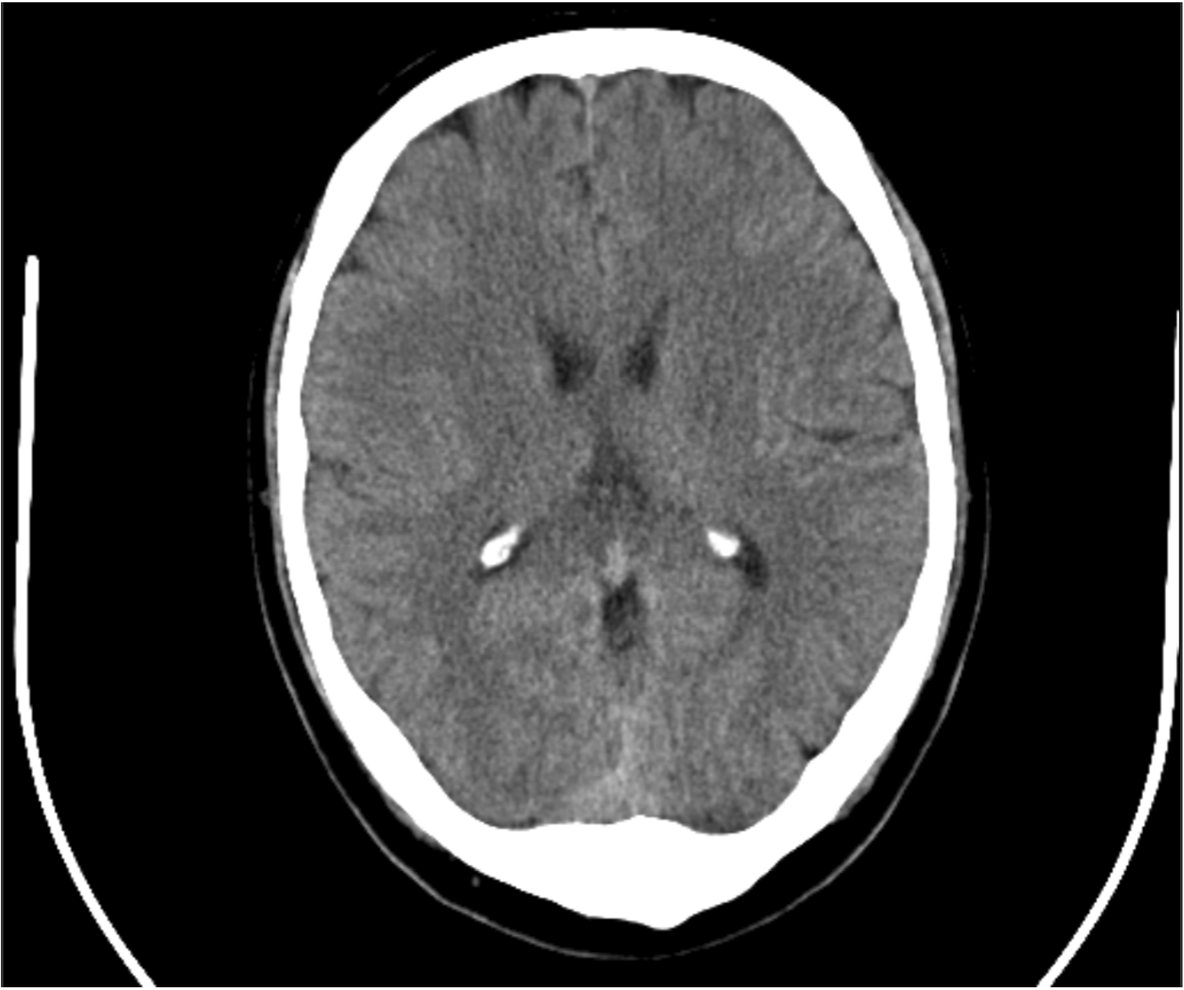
CT before pacemaker implantation
